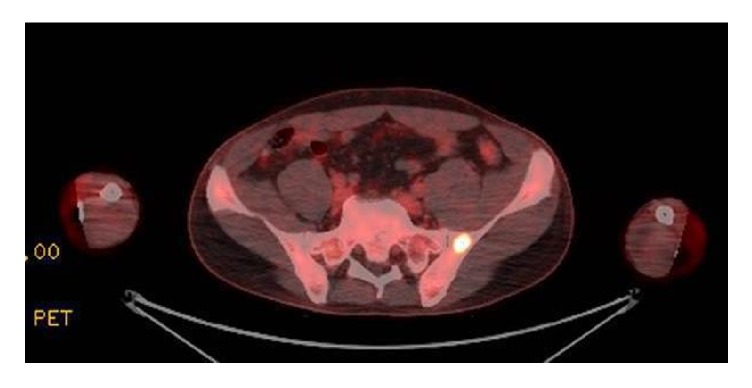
FDG avid lesion in left iliac bone.
